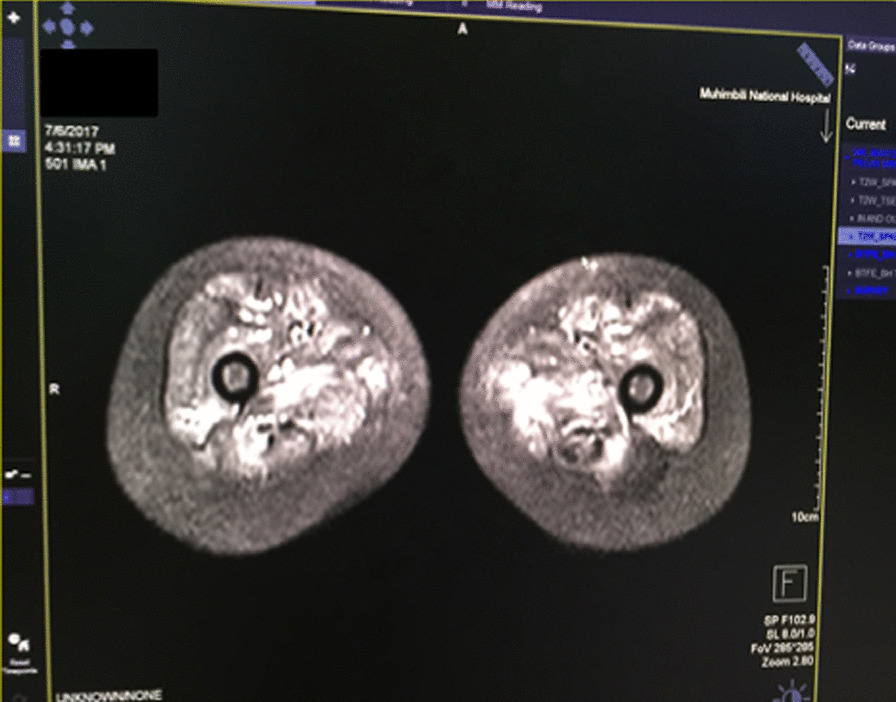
MRI of the proximal legs showing hyperintense signals
